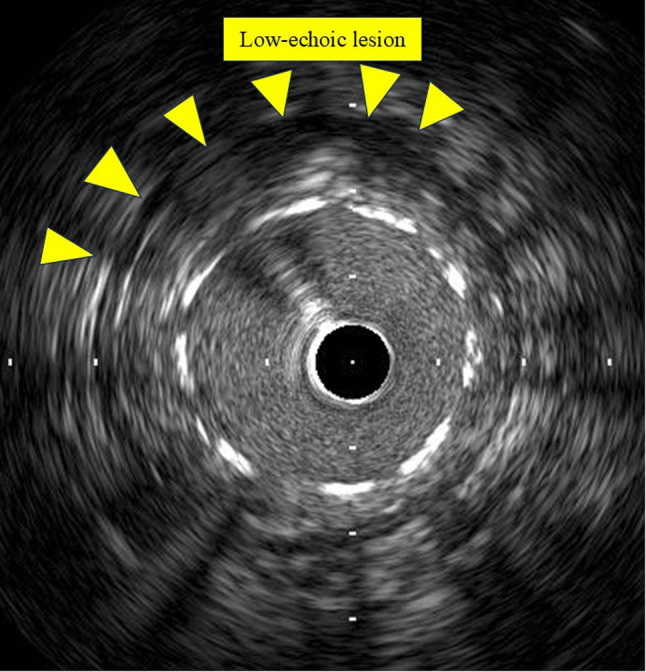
Intravascular ultrasound findings during percutaneous coronary intervention. Intravascular ultrasound obtained during percutaneous coronary intervention demonstrates a circumferential low-echoic lesion surrounding the deployed stent in the right coronary artery (yellow arrowheads). This finding was initially interpreted as thrombus; however, it was subsequently confirmed to represent infected vegetation. The imaging characteristics highlight the difficulty in distinguishing vegetation from thrombotic material in the acute setting
